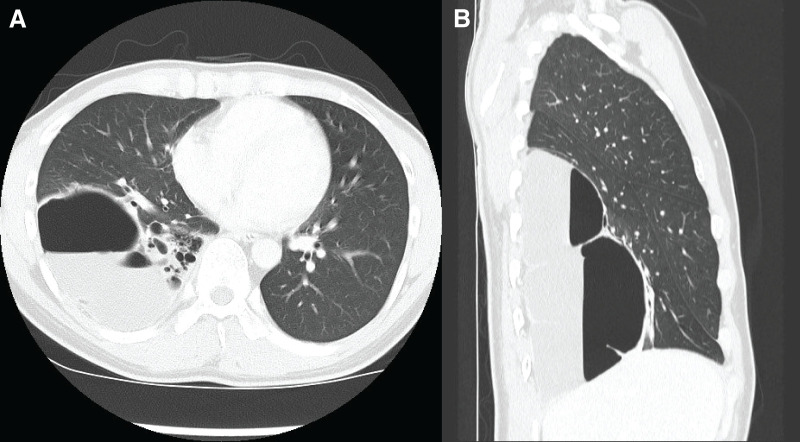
CT image of multiple cystic lesions in the right lower lobe at the age of 26 years. (A) Axial view and (B) sagittal view.
